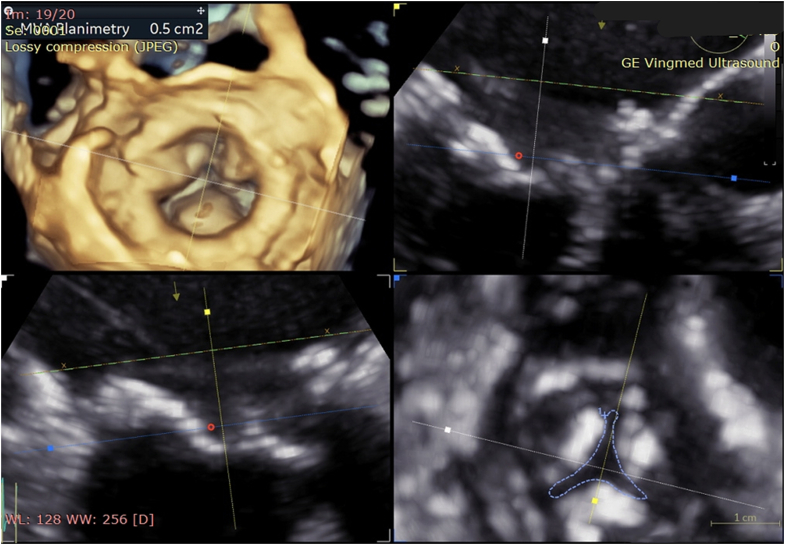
3-Dimensional Transesophageal Echocardiography Showing Severe Bioprosthetic Mitral Stenosis
